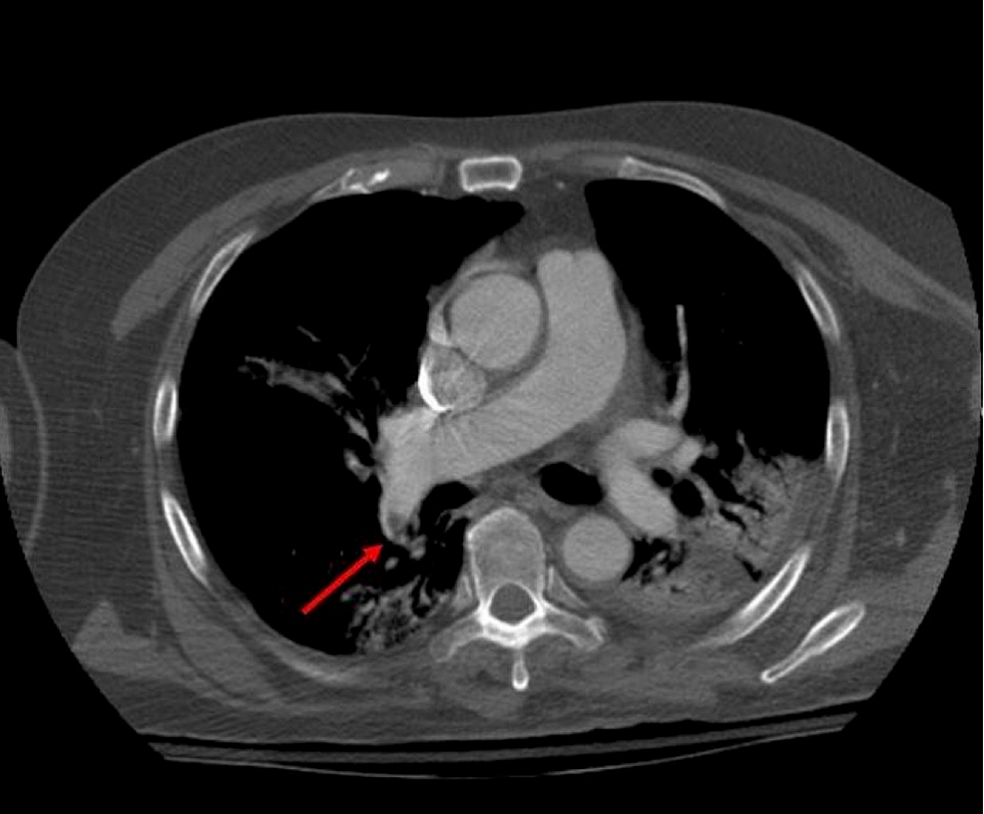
CT of chest depicting a non-occlusive pulmonary emboli (arrow)
